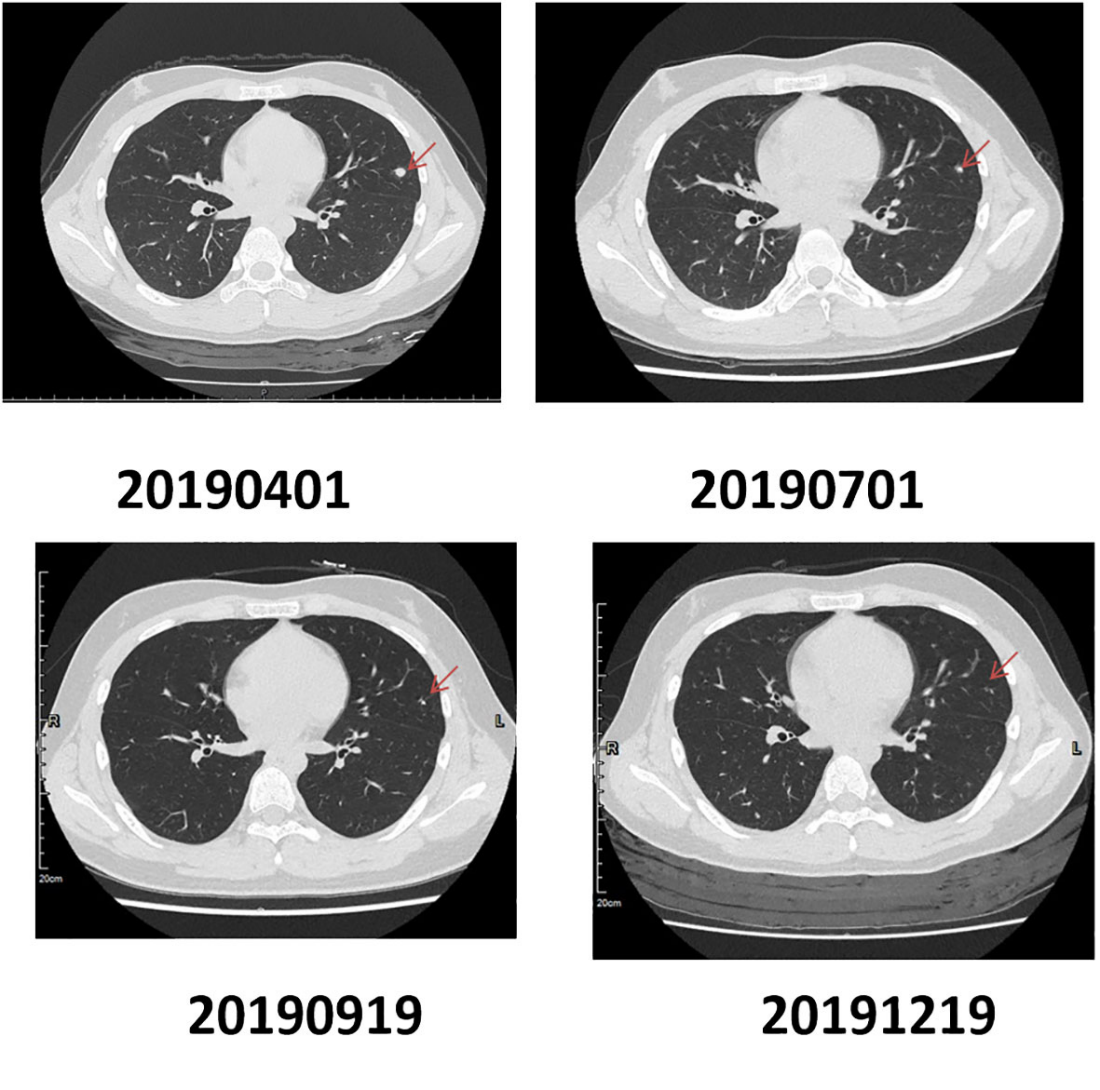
Computed Tomography (CT) imaging changes in tumor size during patient medication regimen.
